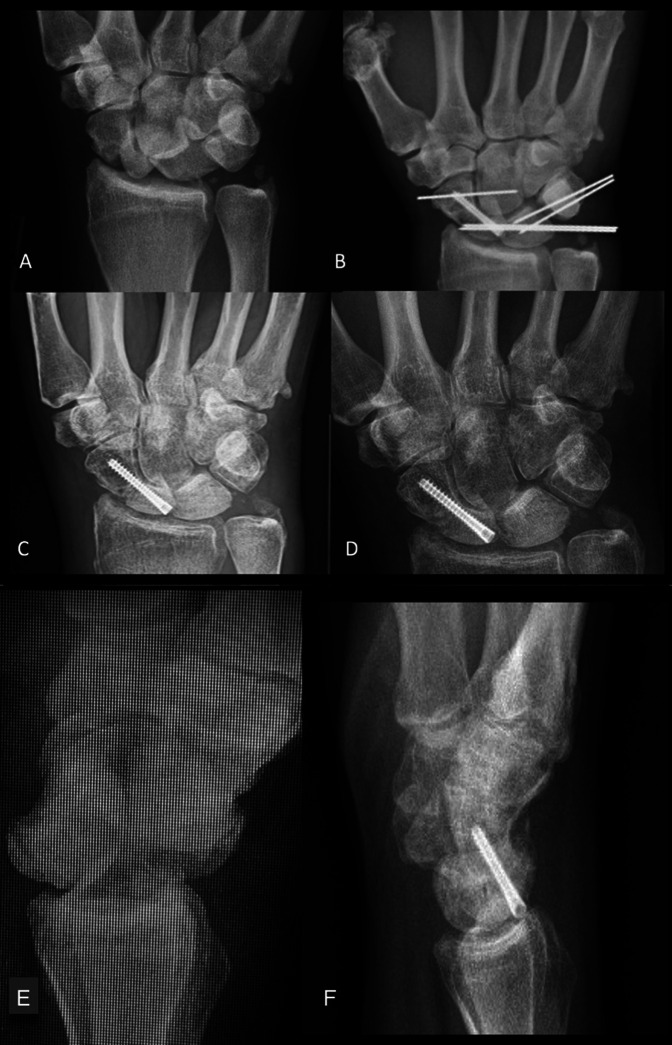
Case 2 serial right wrist, navicular view radiographs demonstrating (A) 4 days post-trauma, (B) postoperative, (C) 22 weeks postoperative, and (D) 1 year postoperative. Note the recession of sclerotic changes between the 22-week and 1-year films indicating neovascularization of the proximal pole of the scaphoid. Lateral wrist radiographs at (E) 4 days and (F) 1 year postoperative.
